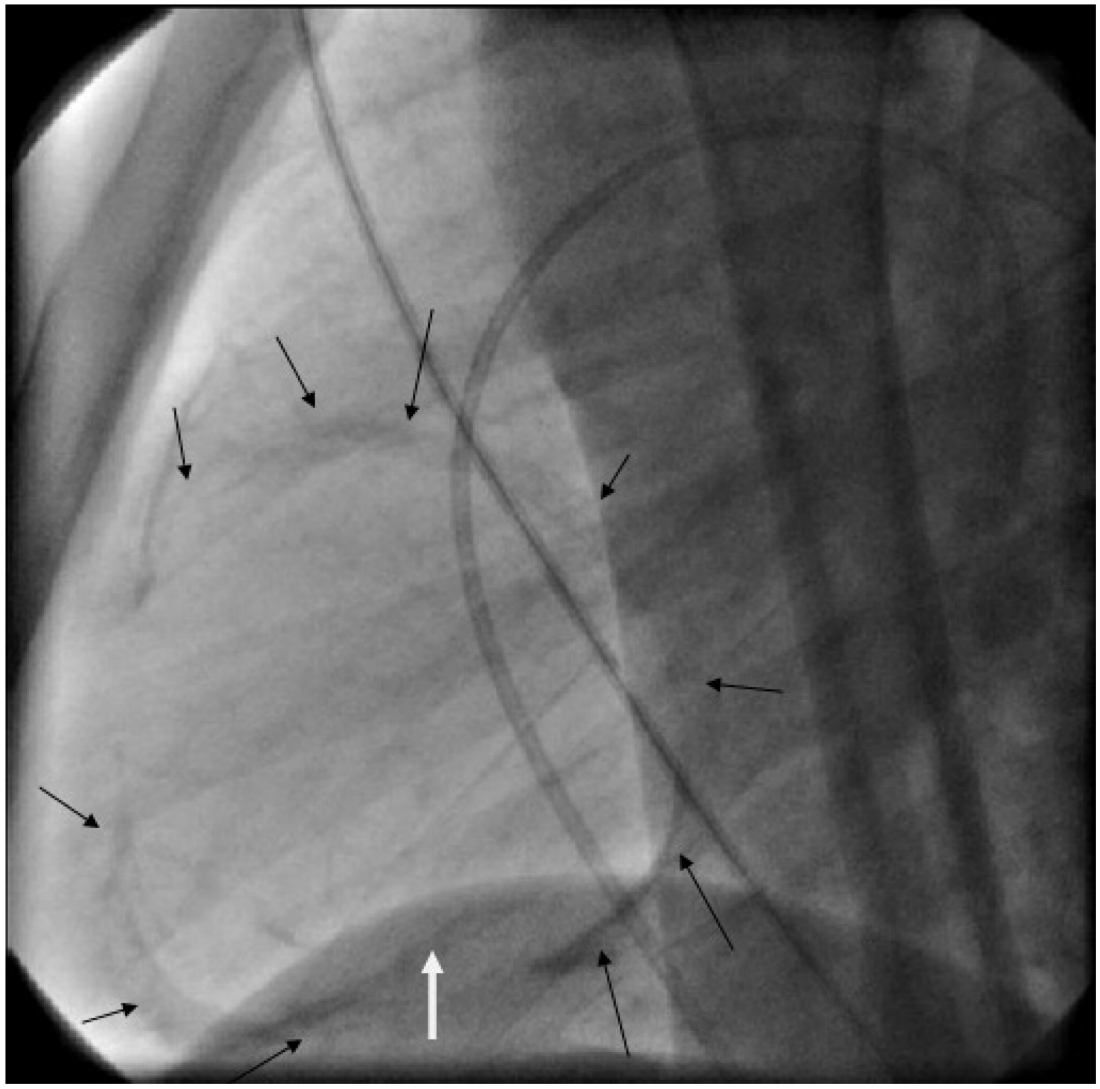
Circumferential calcified band in lateral chest radiograph
